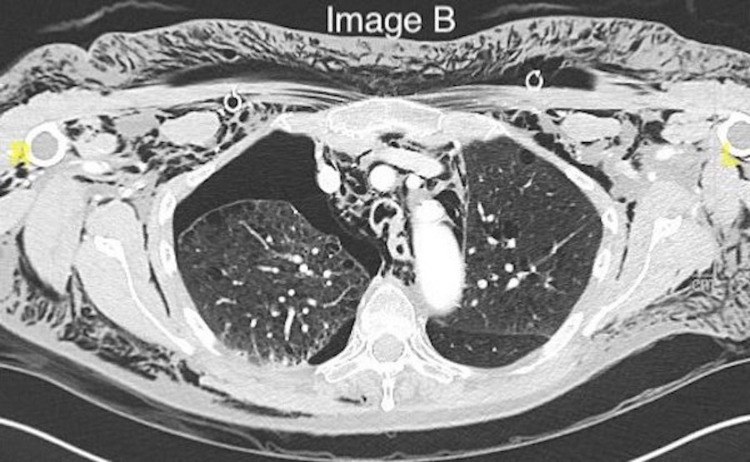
CT thorax demonstrating extensive surgical emphysema, bilateral subcutaneous chest drains, and a right-sided pneumothorax
